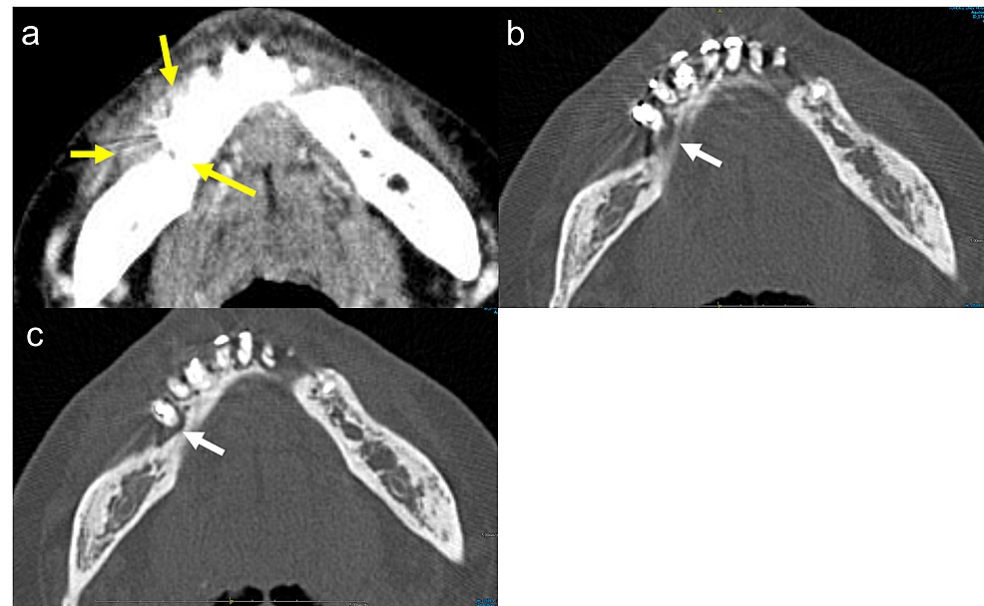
Axial-enhanced CT Axial-enhanced CT scan images of the mandible showed an enhanced tumor that had spread from the right mandibular gingiva to the right lower mandible (yellow arrow) (a). The lesion destroyed the alveolar bone (white arrow) (b). There was also bone resorption around the adjacent teeth (white arrow) (c).
